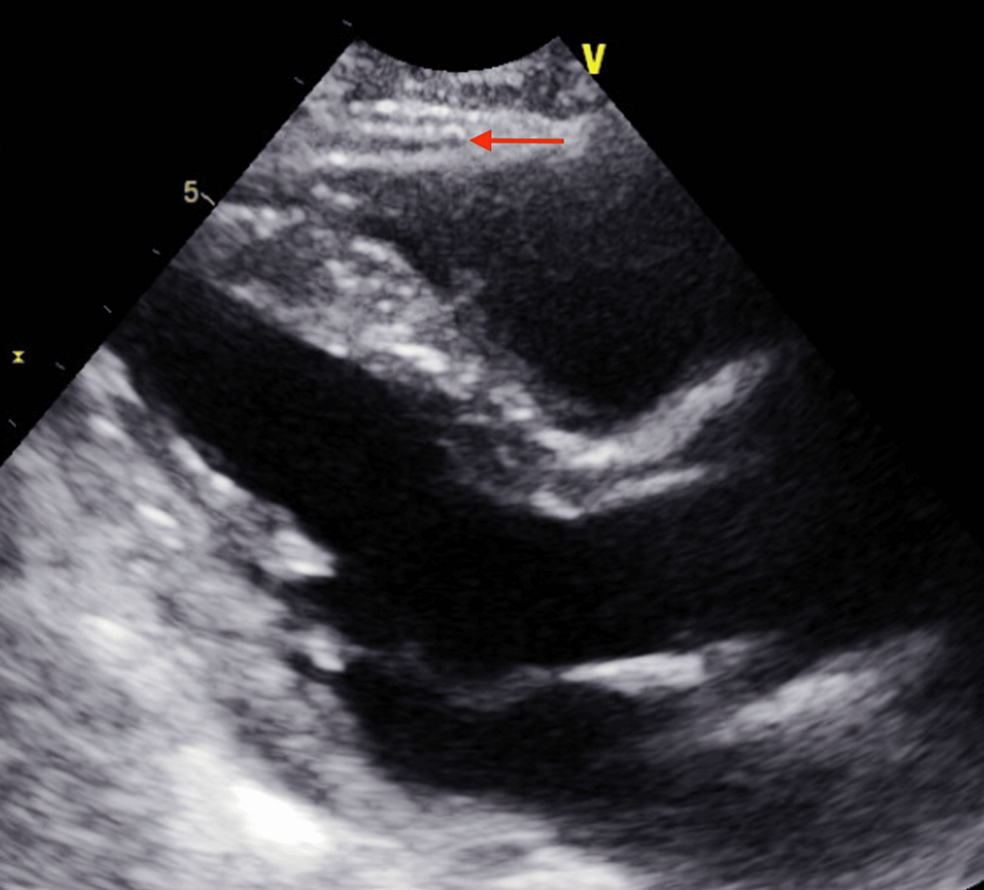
Transthoracic echocardiogram (TTE) after lead removal Transthoracic echocardiogram (TTE) long axis parasternal view after removal of the right ventricular lead shows minimal pericardial effusion and about the same amount prior to lead removal.
